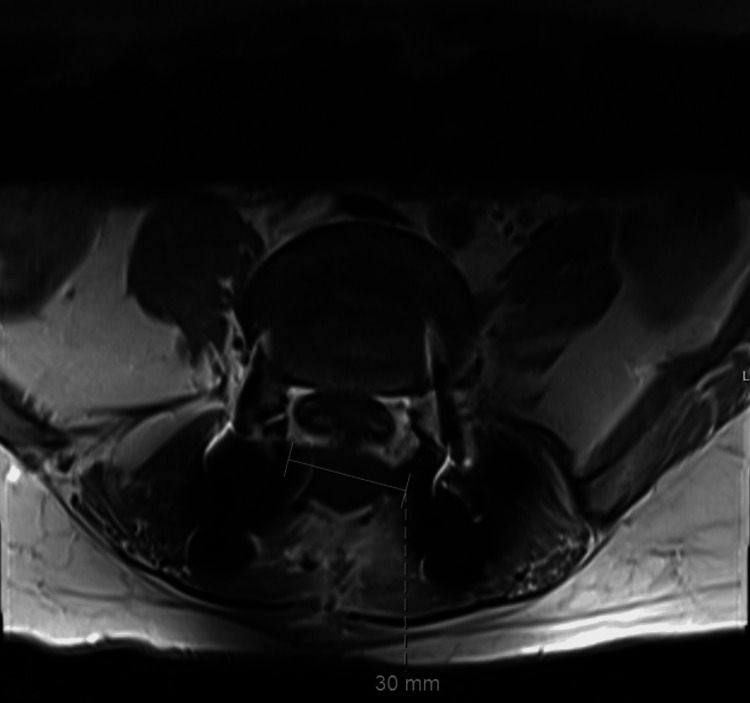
Follow-up MRI lumbar T1 axial demonstrating a 30 mm peripherally enhancing fluid collection along the surgical defect at the native abdominal location.  Fluid accumulation is labeled with the measuring tool.
